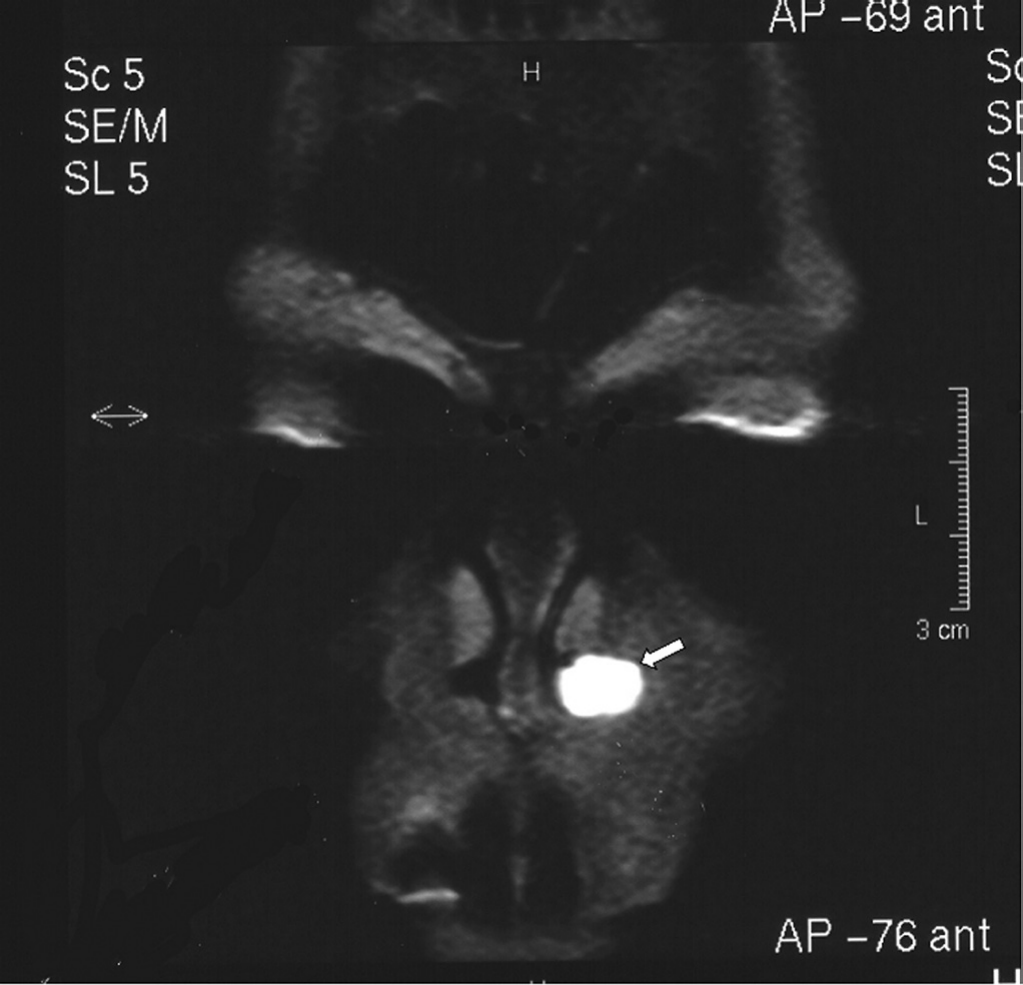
C-) Fat- suppressed MRI image (coronal section). Note hyperintense area in the lower left nasal fossa.
